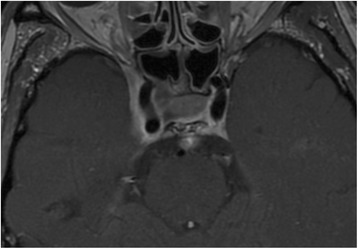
T1-weighted MRI in May of 2017 demonstrating ongoing, subtotal tumor response
